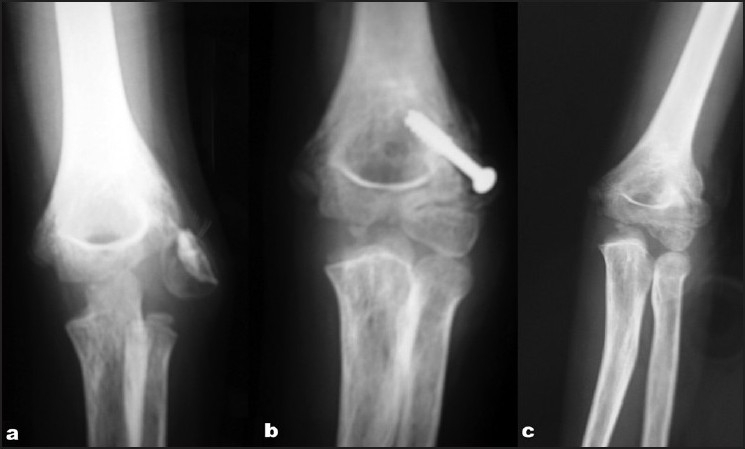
X-ray of the elbow joint (anteroposterior view) showing (a) ten week old fracture of the lateral condyle of the humerus. (b) Less than satisfactory reduction at 8 week follow up, (c) 20 week follow up
